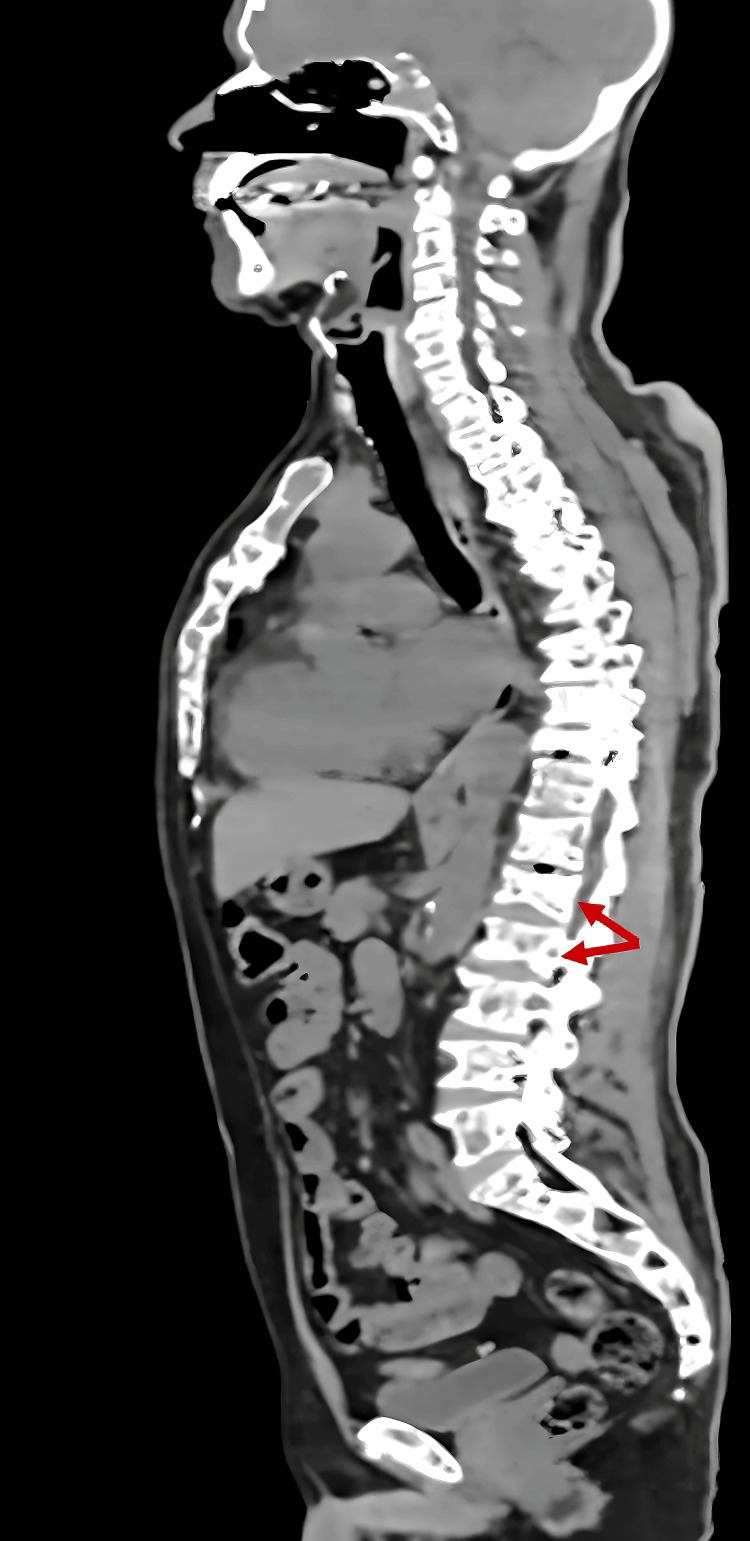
Thoracoabdominal CT scan, sagittal plane, bone window Sagittal CT scan (bone window) of the thoracoabdominal spine demonstrating multiple osteolytic lesions throughout the axial skeleton, with loss of vertebral body height at several levels, most notably at the thoracolumbar junction (arrowhead), suggestive of pathological compression fractures.
